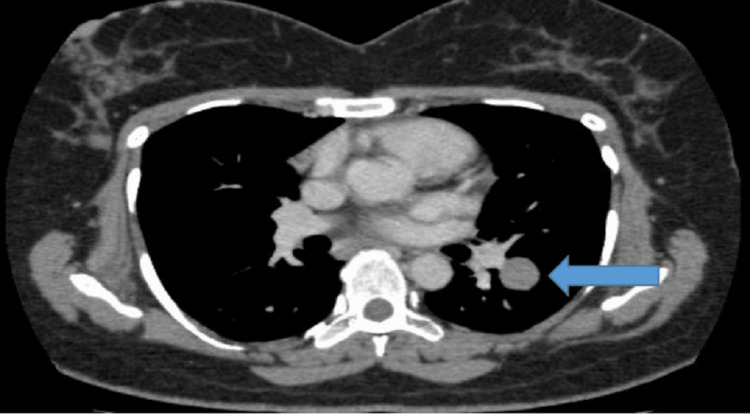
CT of the thorax with contrast (axial view): solitary metastatic deposit of left lower lobe.
